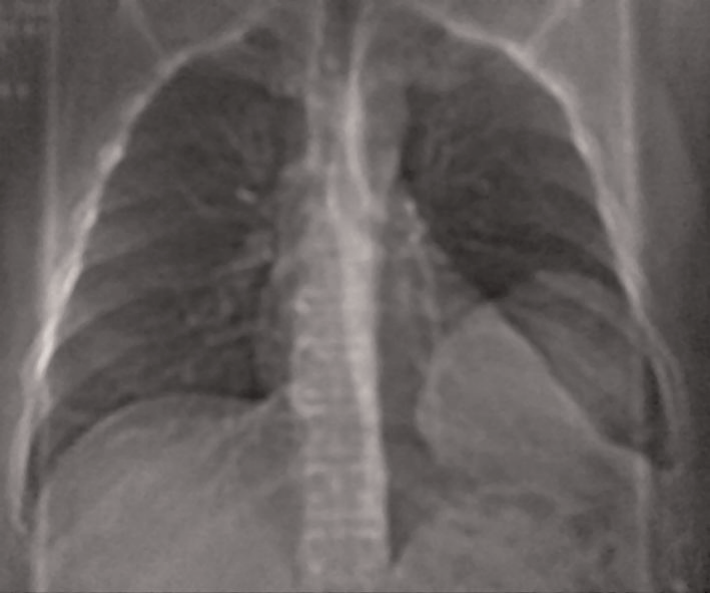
Posteroanterior chest radiograph demonstrates a large, smooth, well-defined mass in the left lower hemithorax.
